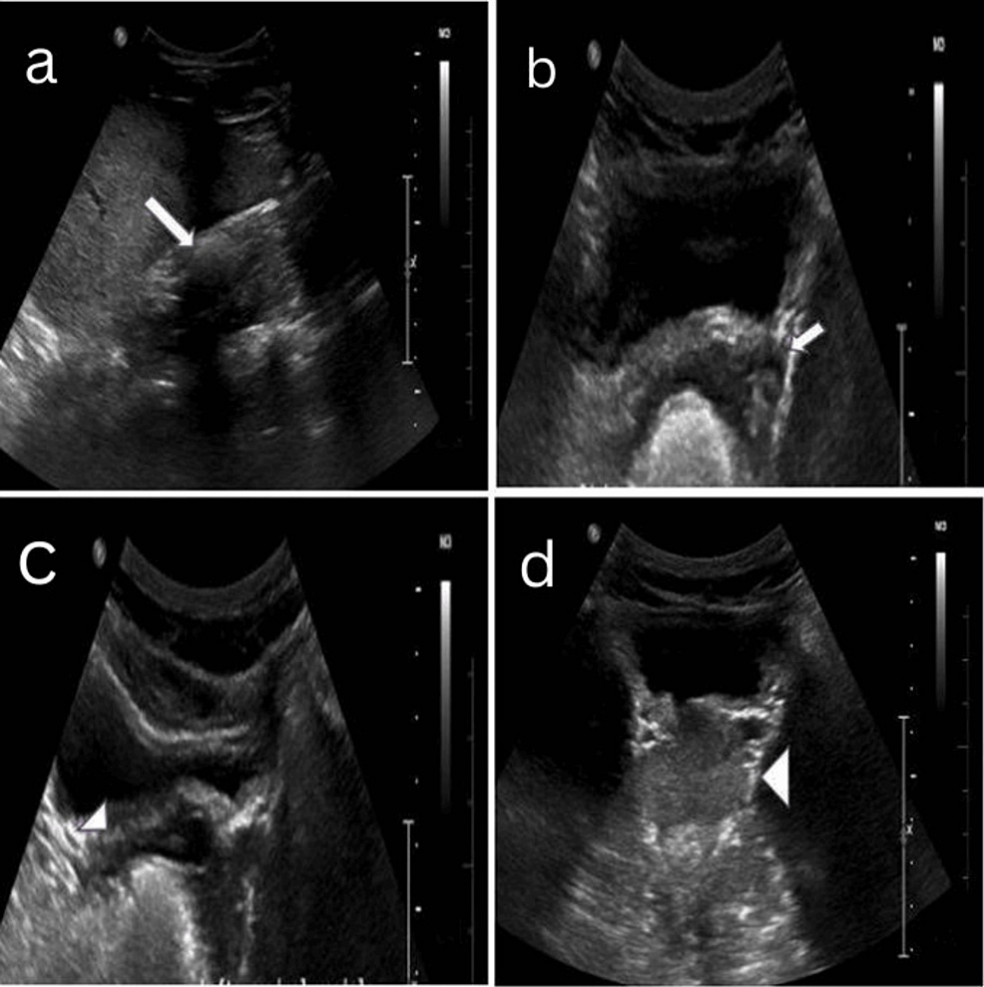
A 21-year-old male presented with a history of recurrent lower urinary tract infections associated with intermittent hematospermia over the past six months. (a) USG of the abdomen (longitudinal and transverse views) shows non-visualization (absence/agenesis) of the left kidney in the left lumbar region (large thick white arrow); (b) left seminal vesicle was enlarged with cystic changes (small thick white arrow); (c) right seminal vesicle was normal (small white arrowhead); and (d) prostate was normal (large white arrowhead). USG: Ultrasonography
